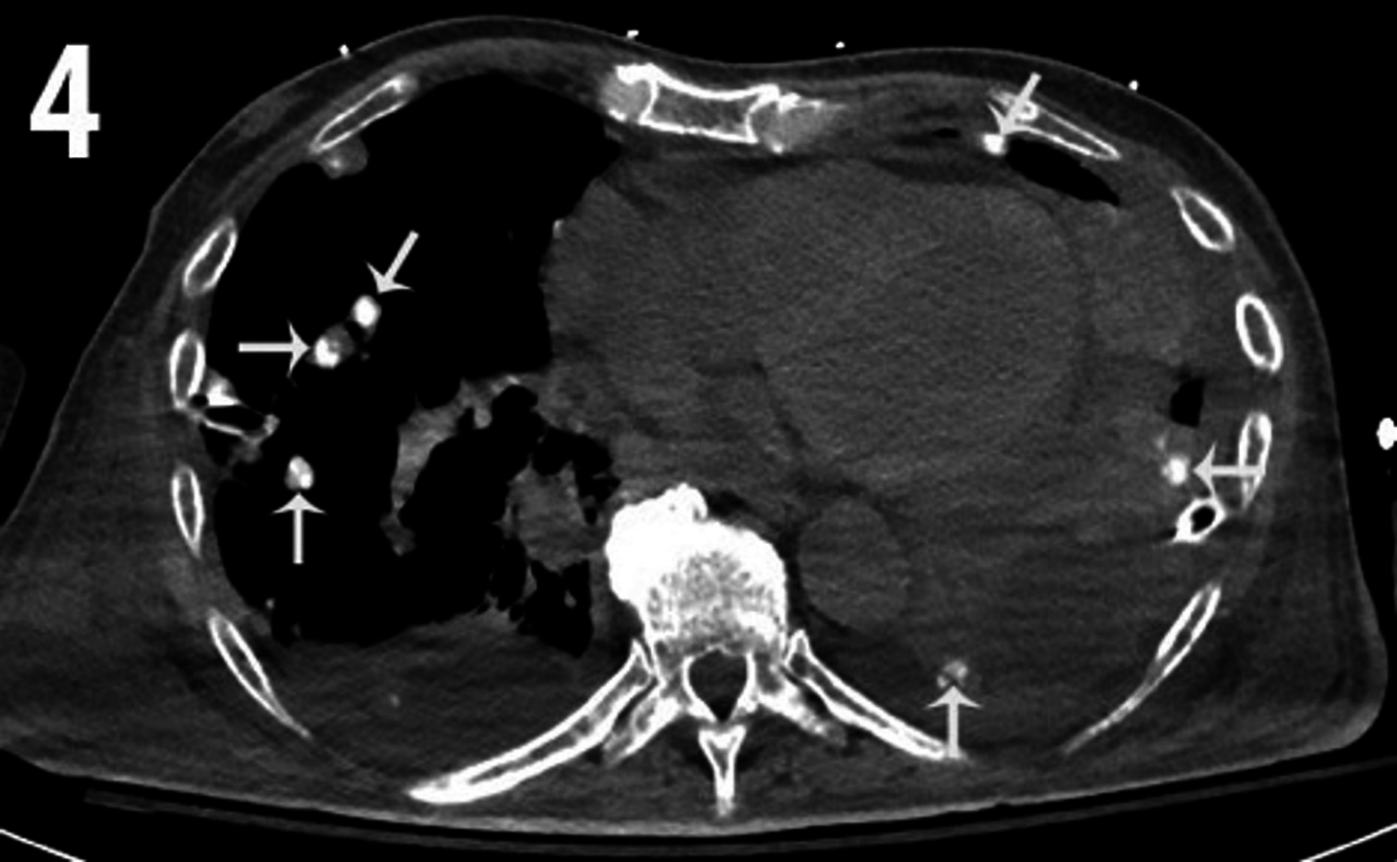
Axial CT image of the chest using soft tissue window levels. Multiple calcified bilateral lung nodules (white arrows). There are also noncalcified, pleural masses in the left cardiophrenic angle. Incidental note of small pericardial and pleural effusions. CT- Computed Tomography
